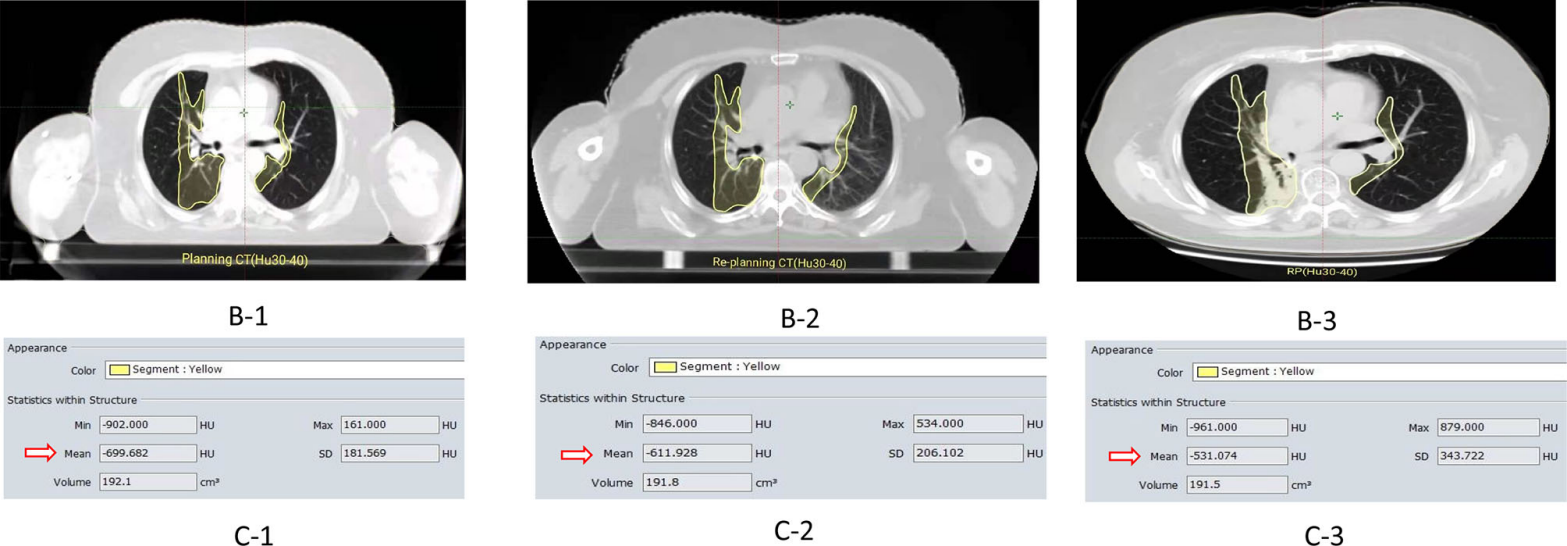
①B-1/-B2 represent the images of the planning CT and the re-planning CT in the HU_30-40_ dose gradient(the yellow curve),respectively. B-3 is the lung image of radiation pneumonia after radiotherapy;②C-1/C-2/C-3 represent CT density values(Hu)in the same or adjacent CT plane in planning CT, re-planning CT, and radiation pneumonia CT respectively (Hu_30-40_); ③ For example, (ΔHU: 87.754)=Planning CT (Hu:-699.682)—Re-planning (Hu:-611.928 Hu).
